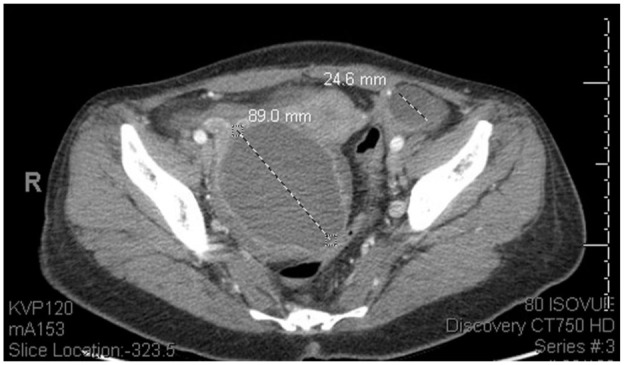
Initial presentation workup: CT abdomen pelvis showing an 8.9 cm complex cystic mass in the region of the right ovary and a 2.4 cm left ovarian cyst.
